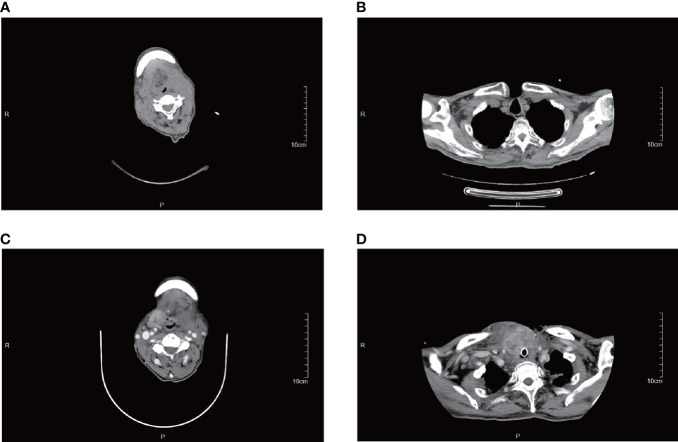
CT images of patient #12. (A, B) are from August 2019, and (C, D) are from December 2019. This patient suffered a rapid enlargement of the right upper cervical and mediastinal lymph nodes in just 4 months and died in December 2019.
